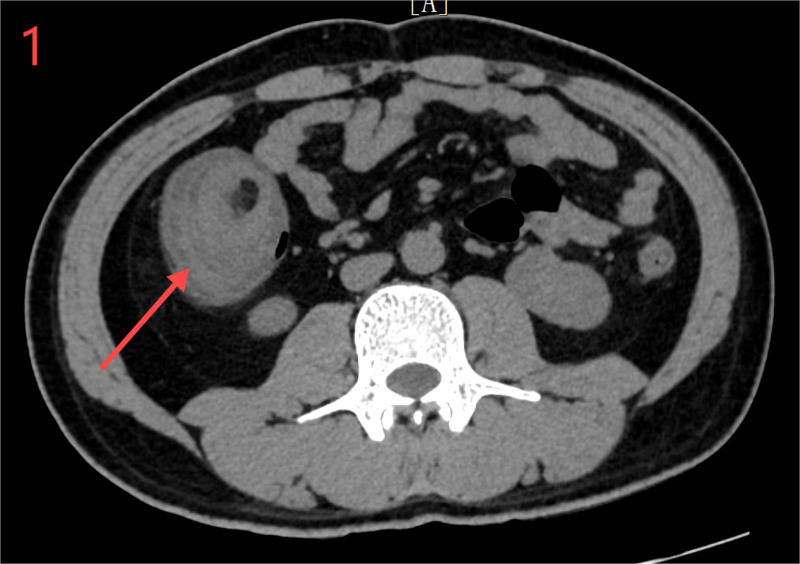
Computed tomography image (transverse plane) shows concentric circle sign in ascending colon (red arrow).
